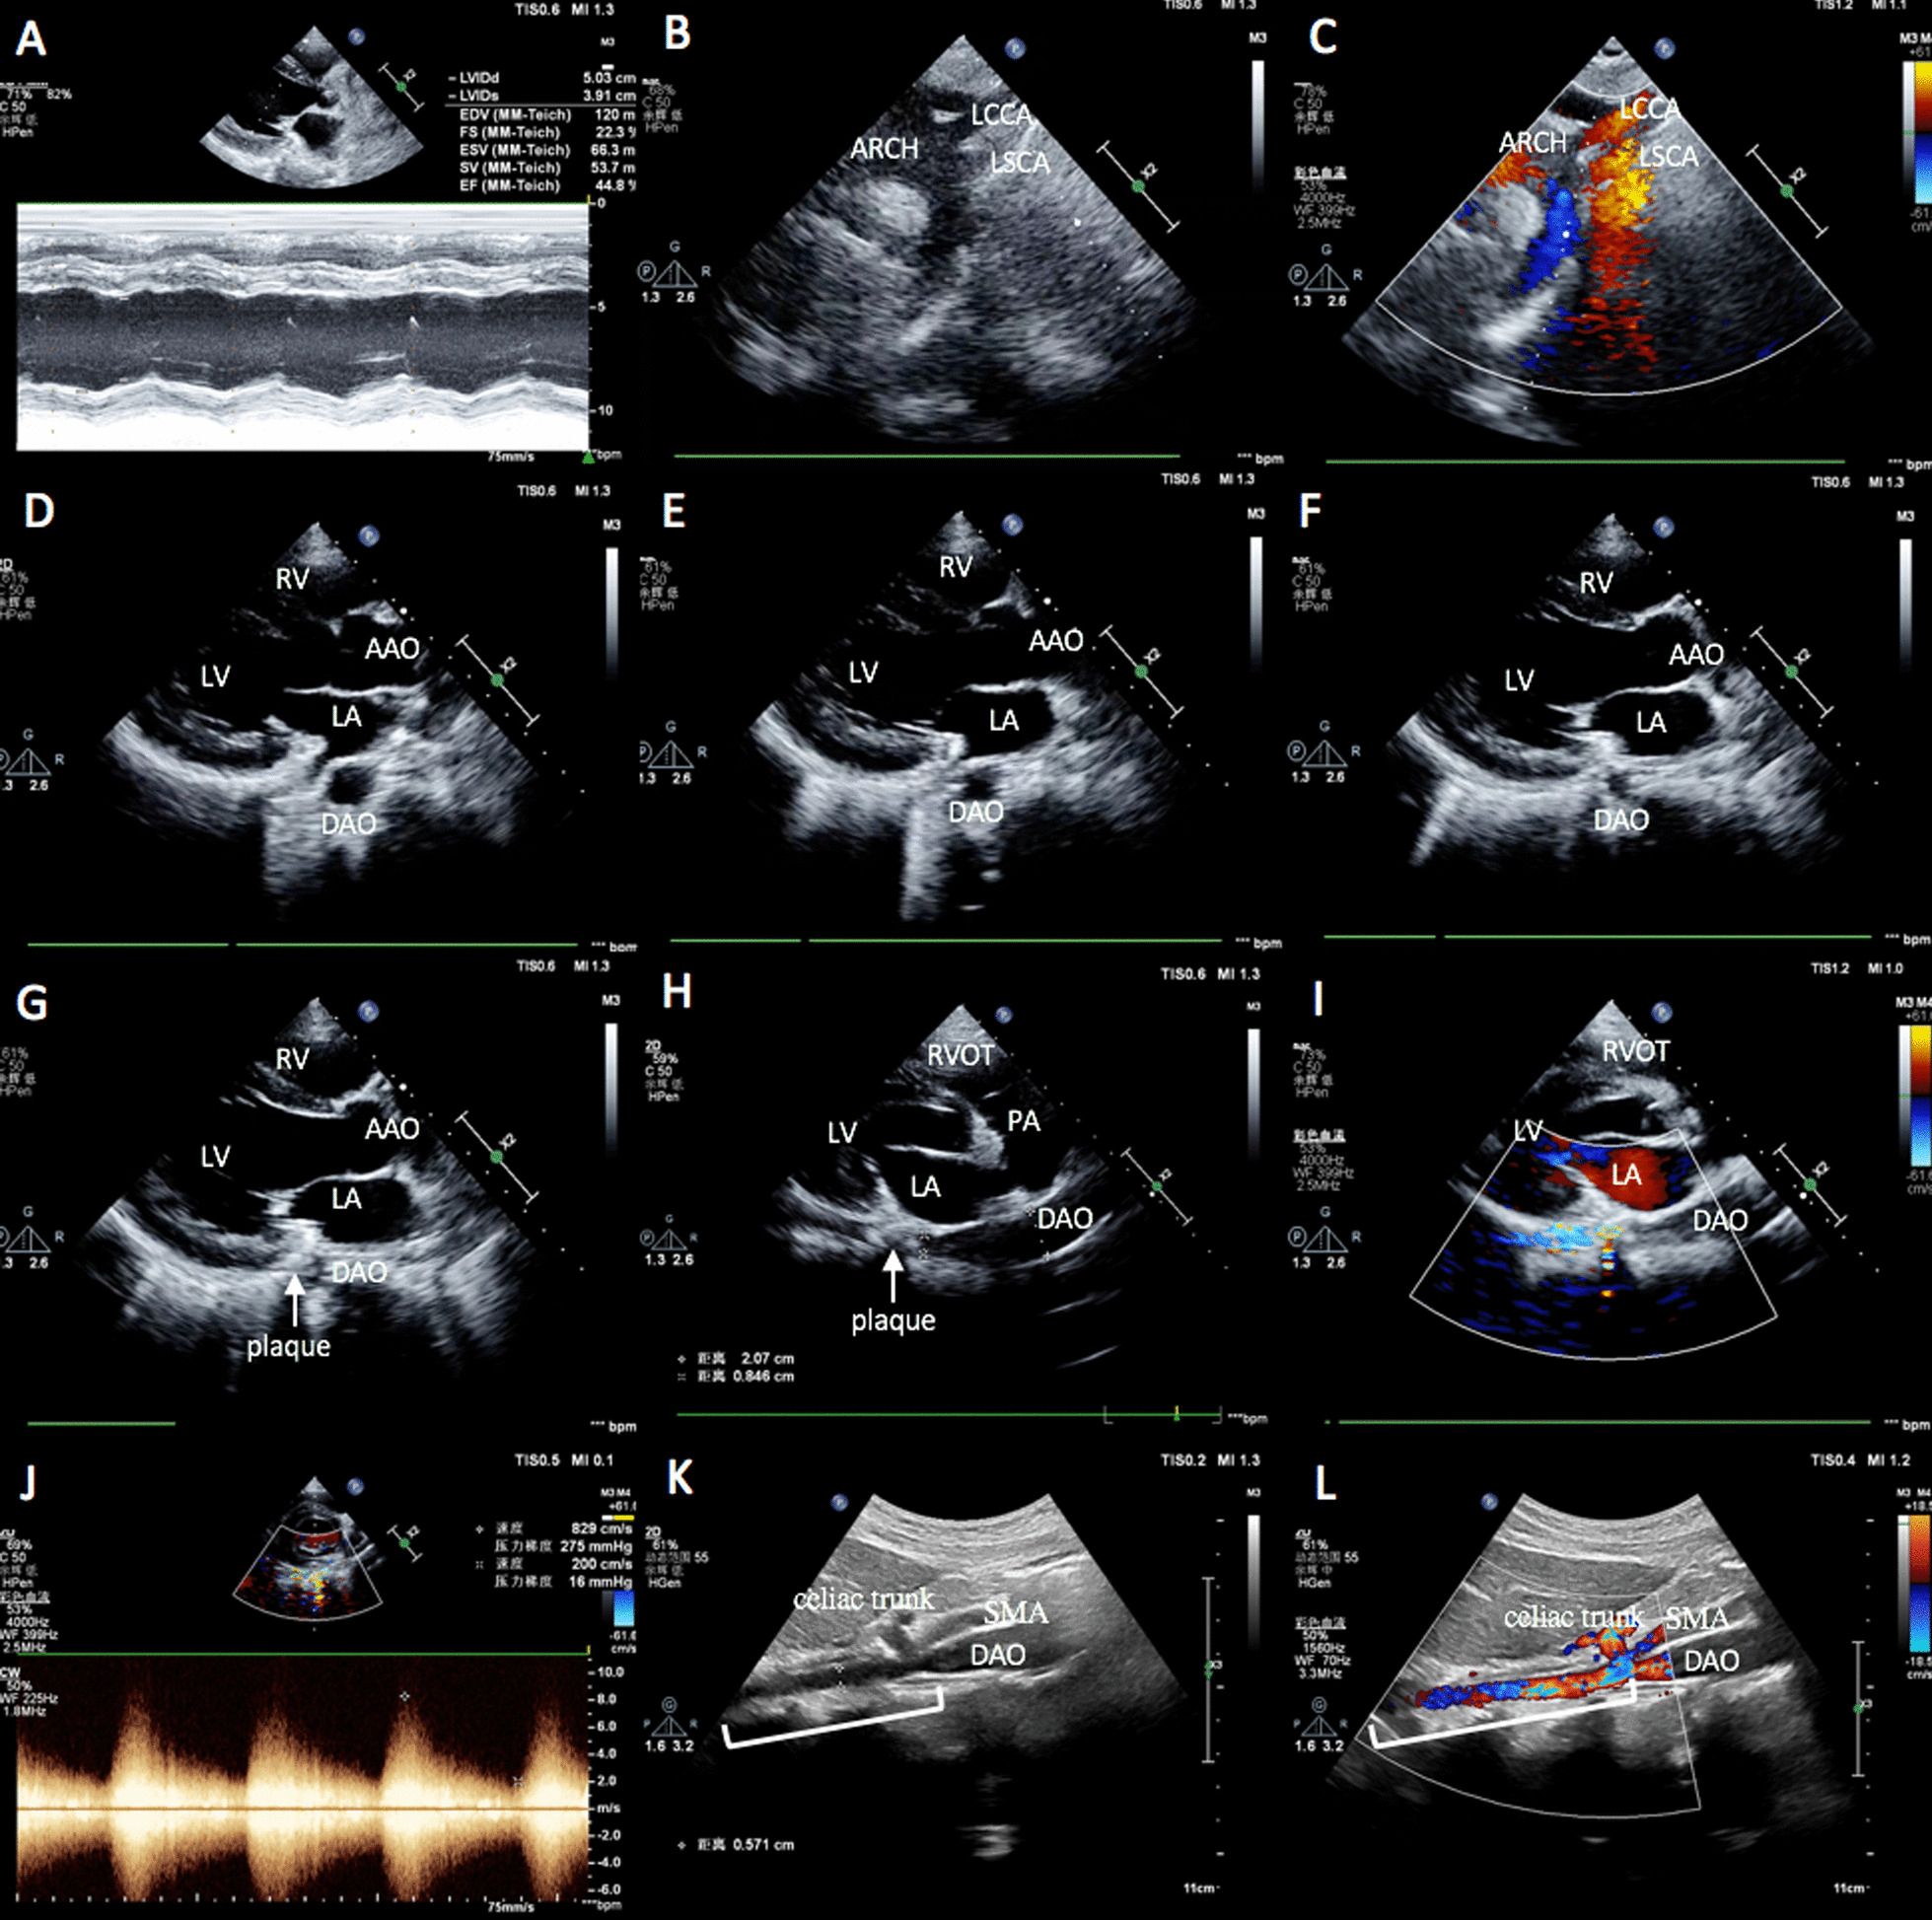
TTE and abdominal vascular ultrasound showed long segmental middle aortic coarctation. A M mode showed the left ventricular hypertrophy with a reduced ejection fraction to 44.8%. B, C The suprasternal view showed no aortic coarctation in the isthmus. D–G With a slight adjustment of the probe in the parasternal long-axis view of the left ventricle, the diameter of the thoracic descending aorta behind the left atrium gradually decreased. H–J The longitudinal axis of the thoracic descending aorta showed a segmental narrowing with a calcified plaque(arrow) in the thoracic descending aorta below the level of the atrioventricular sulcus, Color Doppler showed a turbulent flow in the narrowing segment with a peak velocity of 829 cm/s. K–L Abdominal vascular ultrasound showed a long segment narrowing (bracket) of descending abdominal aorta above the level of the superior mesenteric artery. *LV* left ventricle, *LA* left atrium, *RVOT* right ventricular outflow tract, *RV* right ventricle, *SMA* superior mesenteric artery, *AAO* ascending aorta, *DAO* descending aorta, *ARCH* aortic arch, *LCCA* left common carotid artery, *LSCA* left subclavian artery
